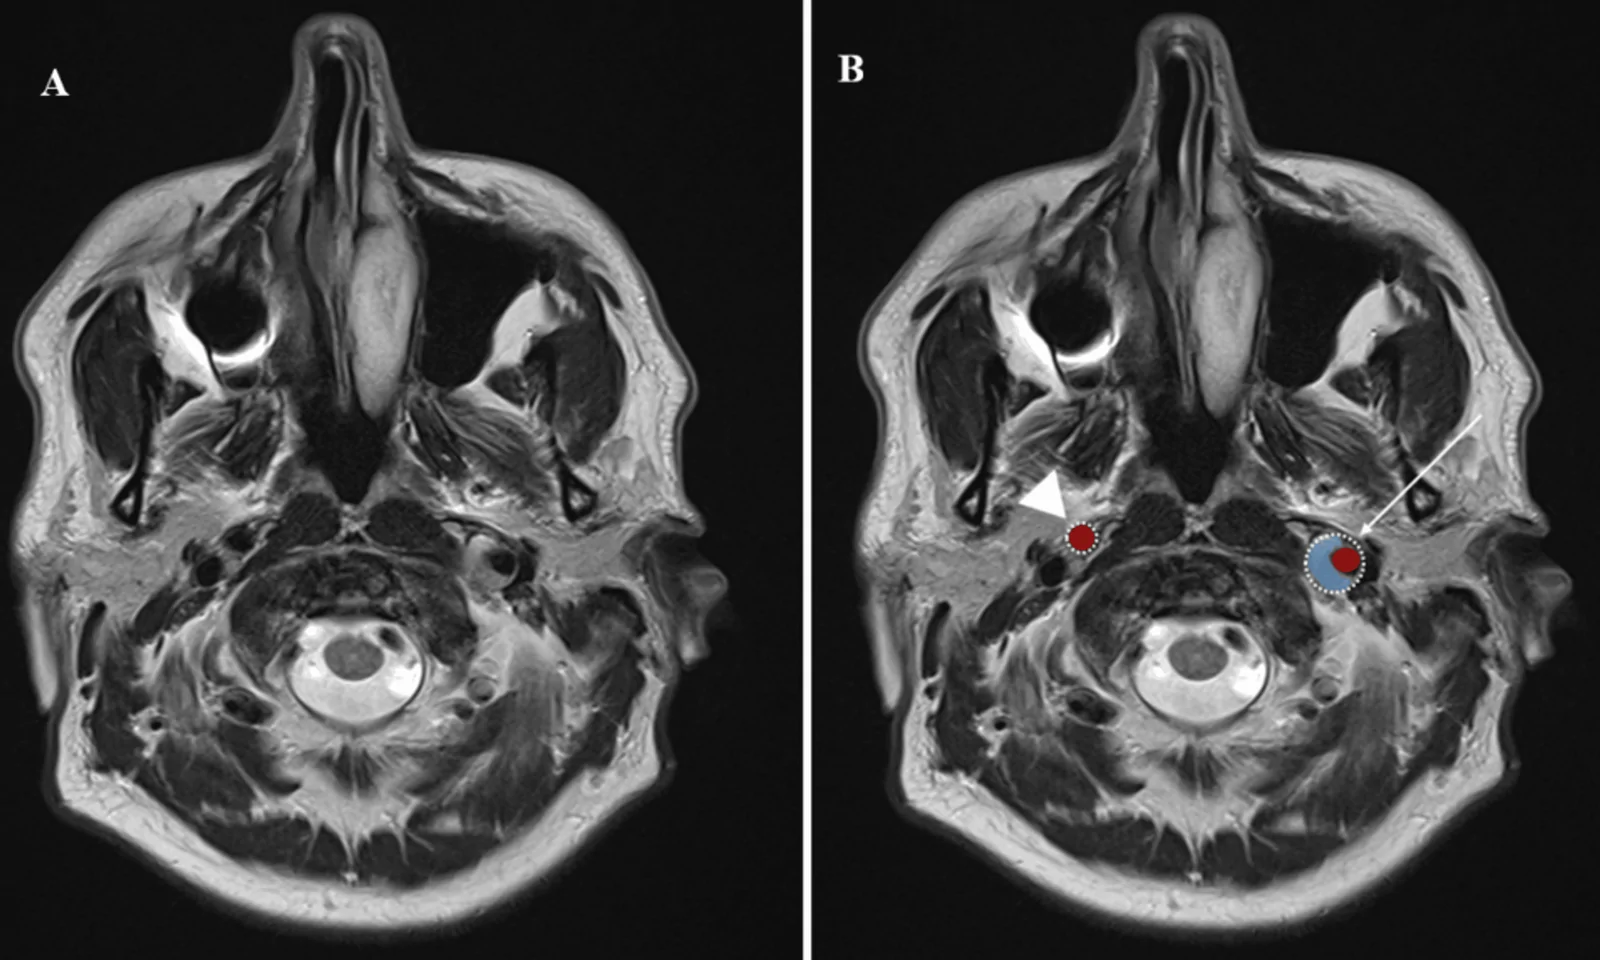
Axial T2-weighted MRI (A) and annotated image (B) at a level below the skull base showing the distal right (arrowhead) and left (arrow) internal carotid arteries. The left ICA diameter is expanded (dotted line) due to an intramural hematoma (blue area), which is easily identified by the signal contrast between the T2-hyperintense hematoma and the T2-hypointense flowing blood within the arterial lumen ICA: internal carotid artery; MRI: magnetic resonance imaging
